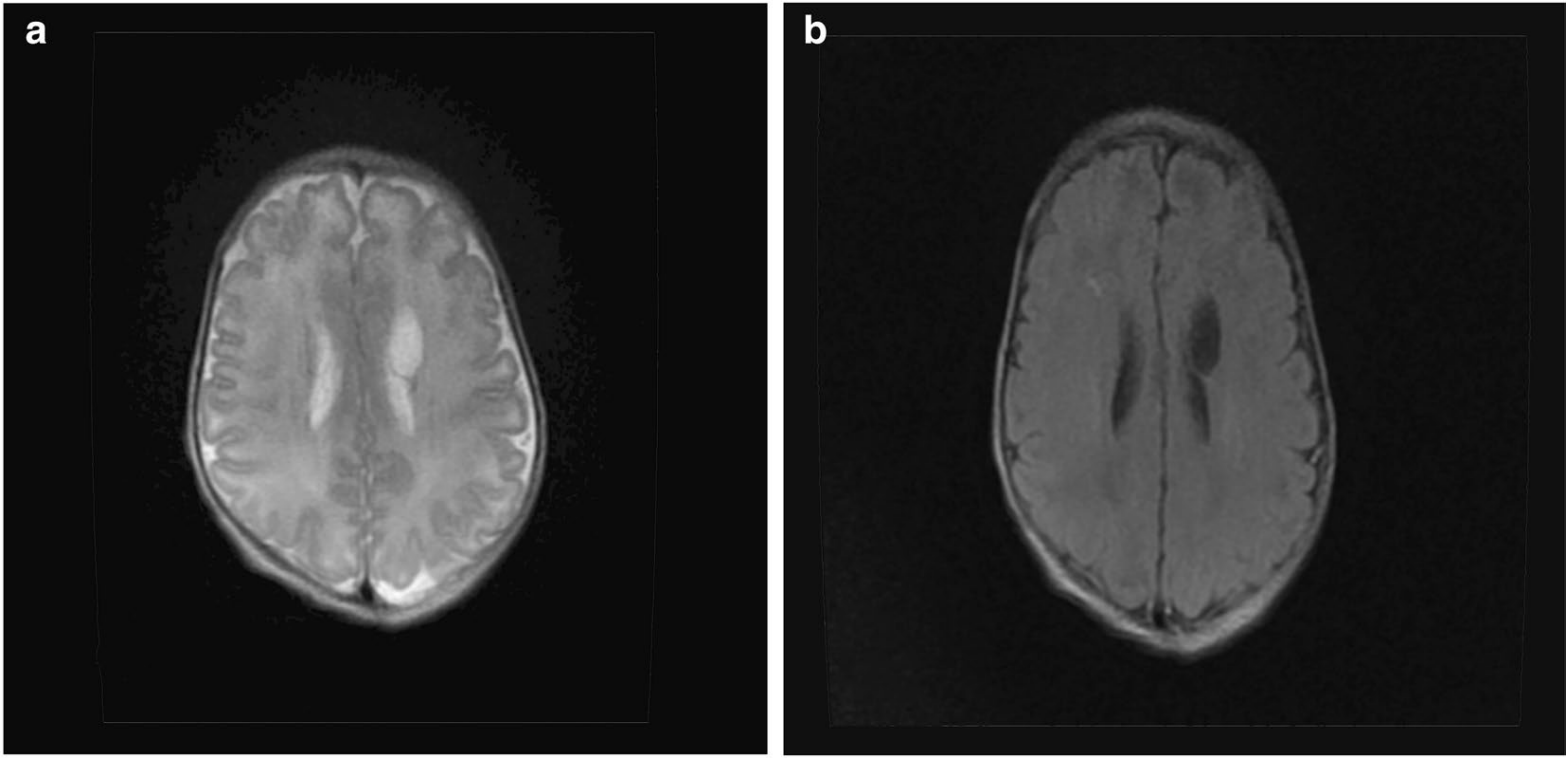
Brain MRI showing a left frontal lobe cyst
